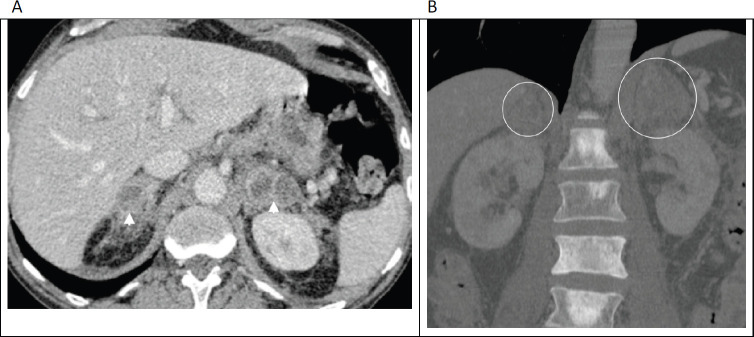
(a): Axial contrast enhanced porto-venous phase abdominal CT demonstrating bilateral, heterogeneously enhancing masses involving the adrenal glands (arrowheads) in a 66-year-old man with known prostatic cancer. (b): Coronal bone window in the same patient shows multiple sclerotic bone metastases, with the enlarged adrenal glands just visible on this window (circled). These adrenal lesions were new/enlarging compared with prior imaging studies and were accompanied by progression of nodal and osseous metastases; leading to diagnosis of adrenal metastases made on clinical grounds.
